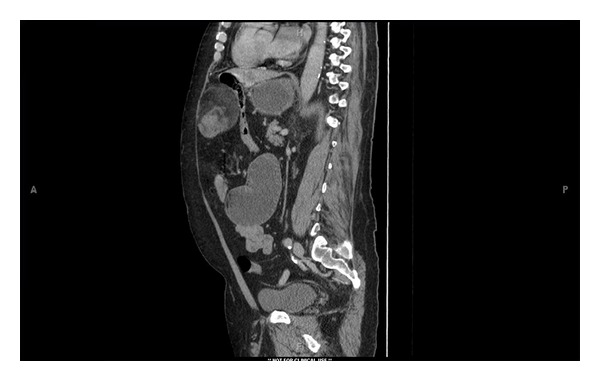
Computed tomographic sagittal image showing anterior epigastric mass compressing distal small bowel, causing dilated duodenum and stomach proximally.
